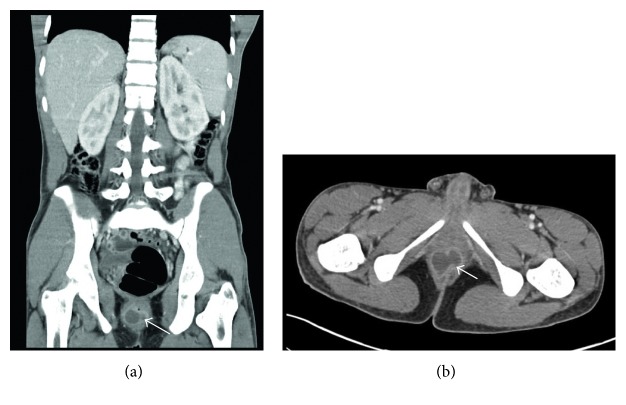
Solid dense arrow delineating perirectal abscess in the saggital (a) and coronal (b) view of the abdominal CT scan.
